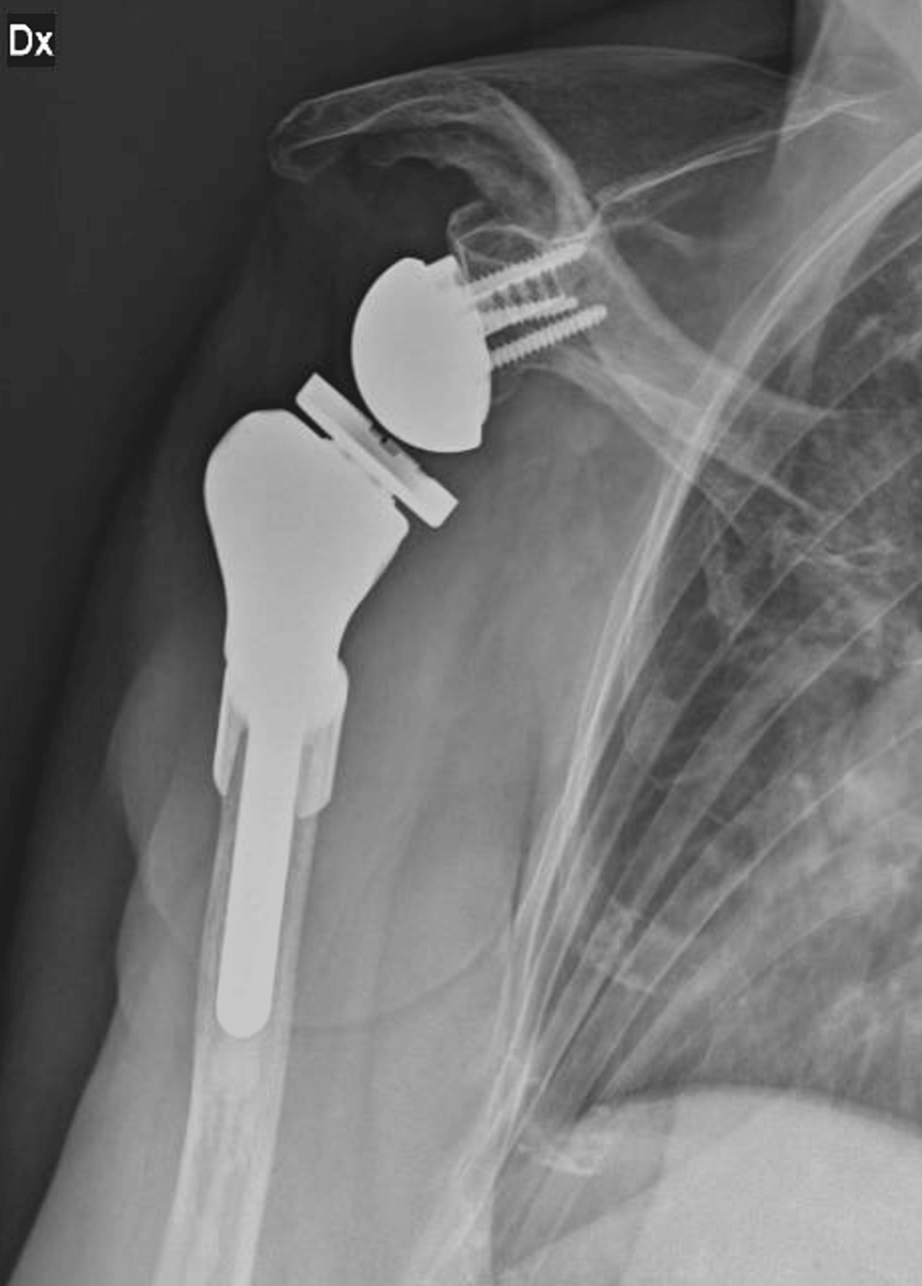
Twelve-month post-megaprosthesis X-ray evaluation (Case 2) Dx: right
